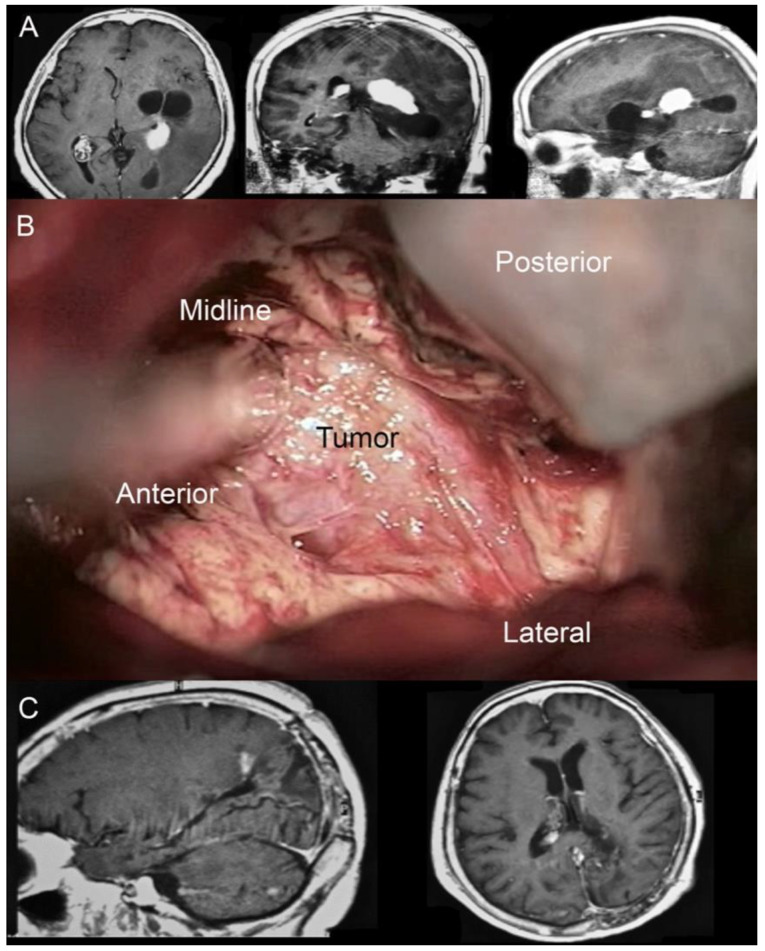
(A) Pre-operative MRI showing a left-sided partially cystic lesion localized in the atrium and partially involving the temporal horn. (B) Intraoperative photograph propaedeutic for videoclip orientation. (C) Postoperative MRI showing complete tumor removal.
